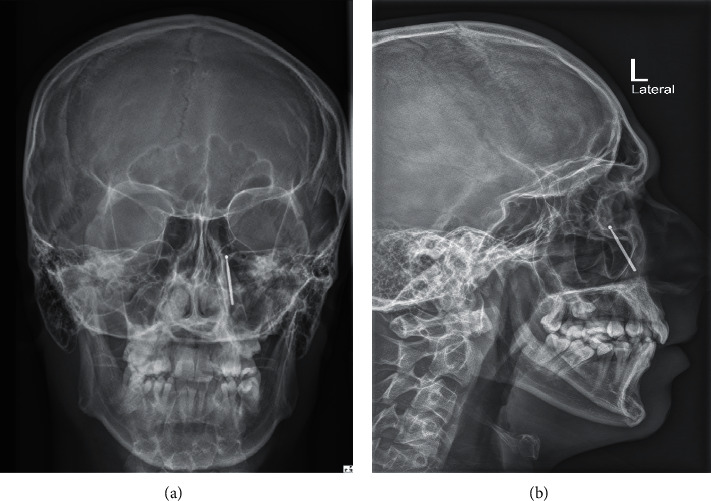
The anterioposterior and lateral view X-ray showing the radio opaque foreign body situated in the left maxillary sinus with head of the foreign body pointing posteriorly.
